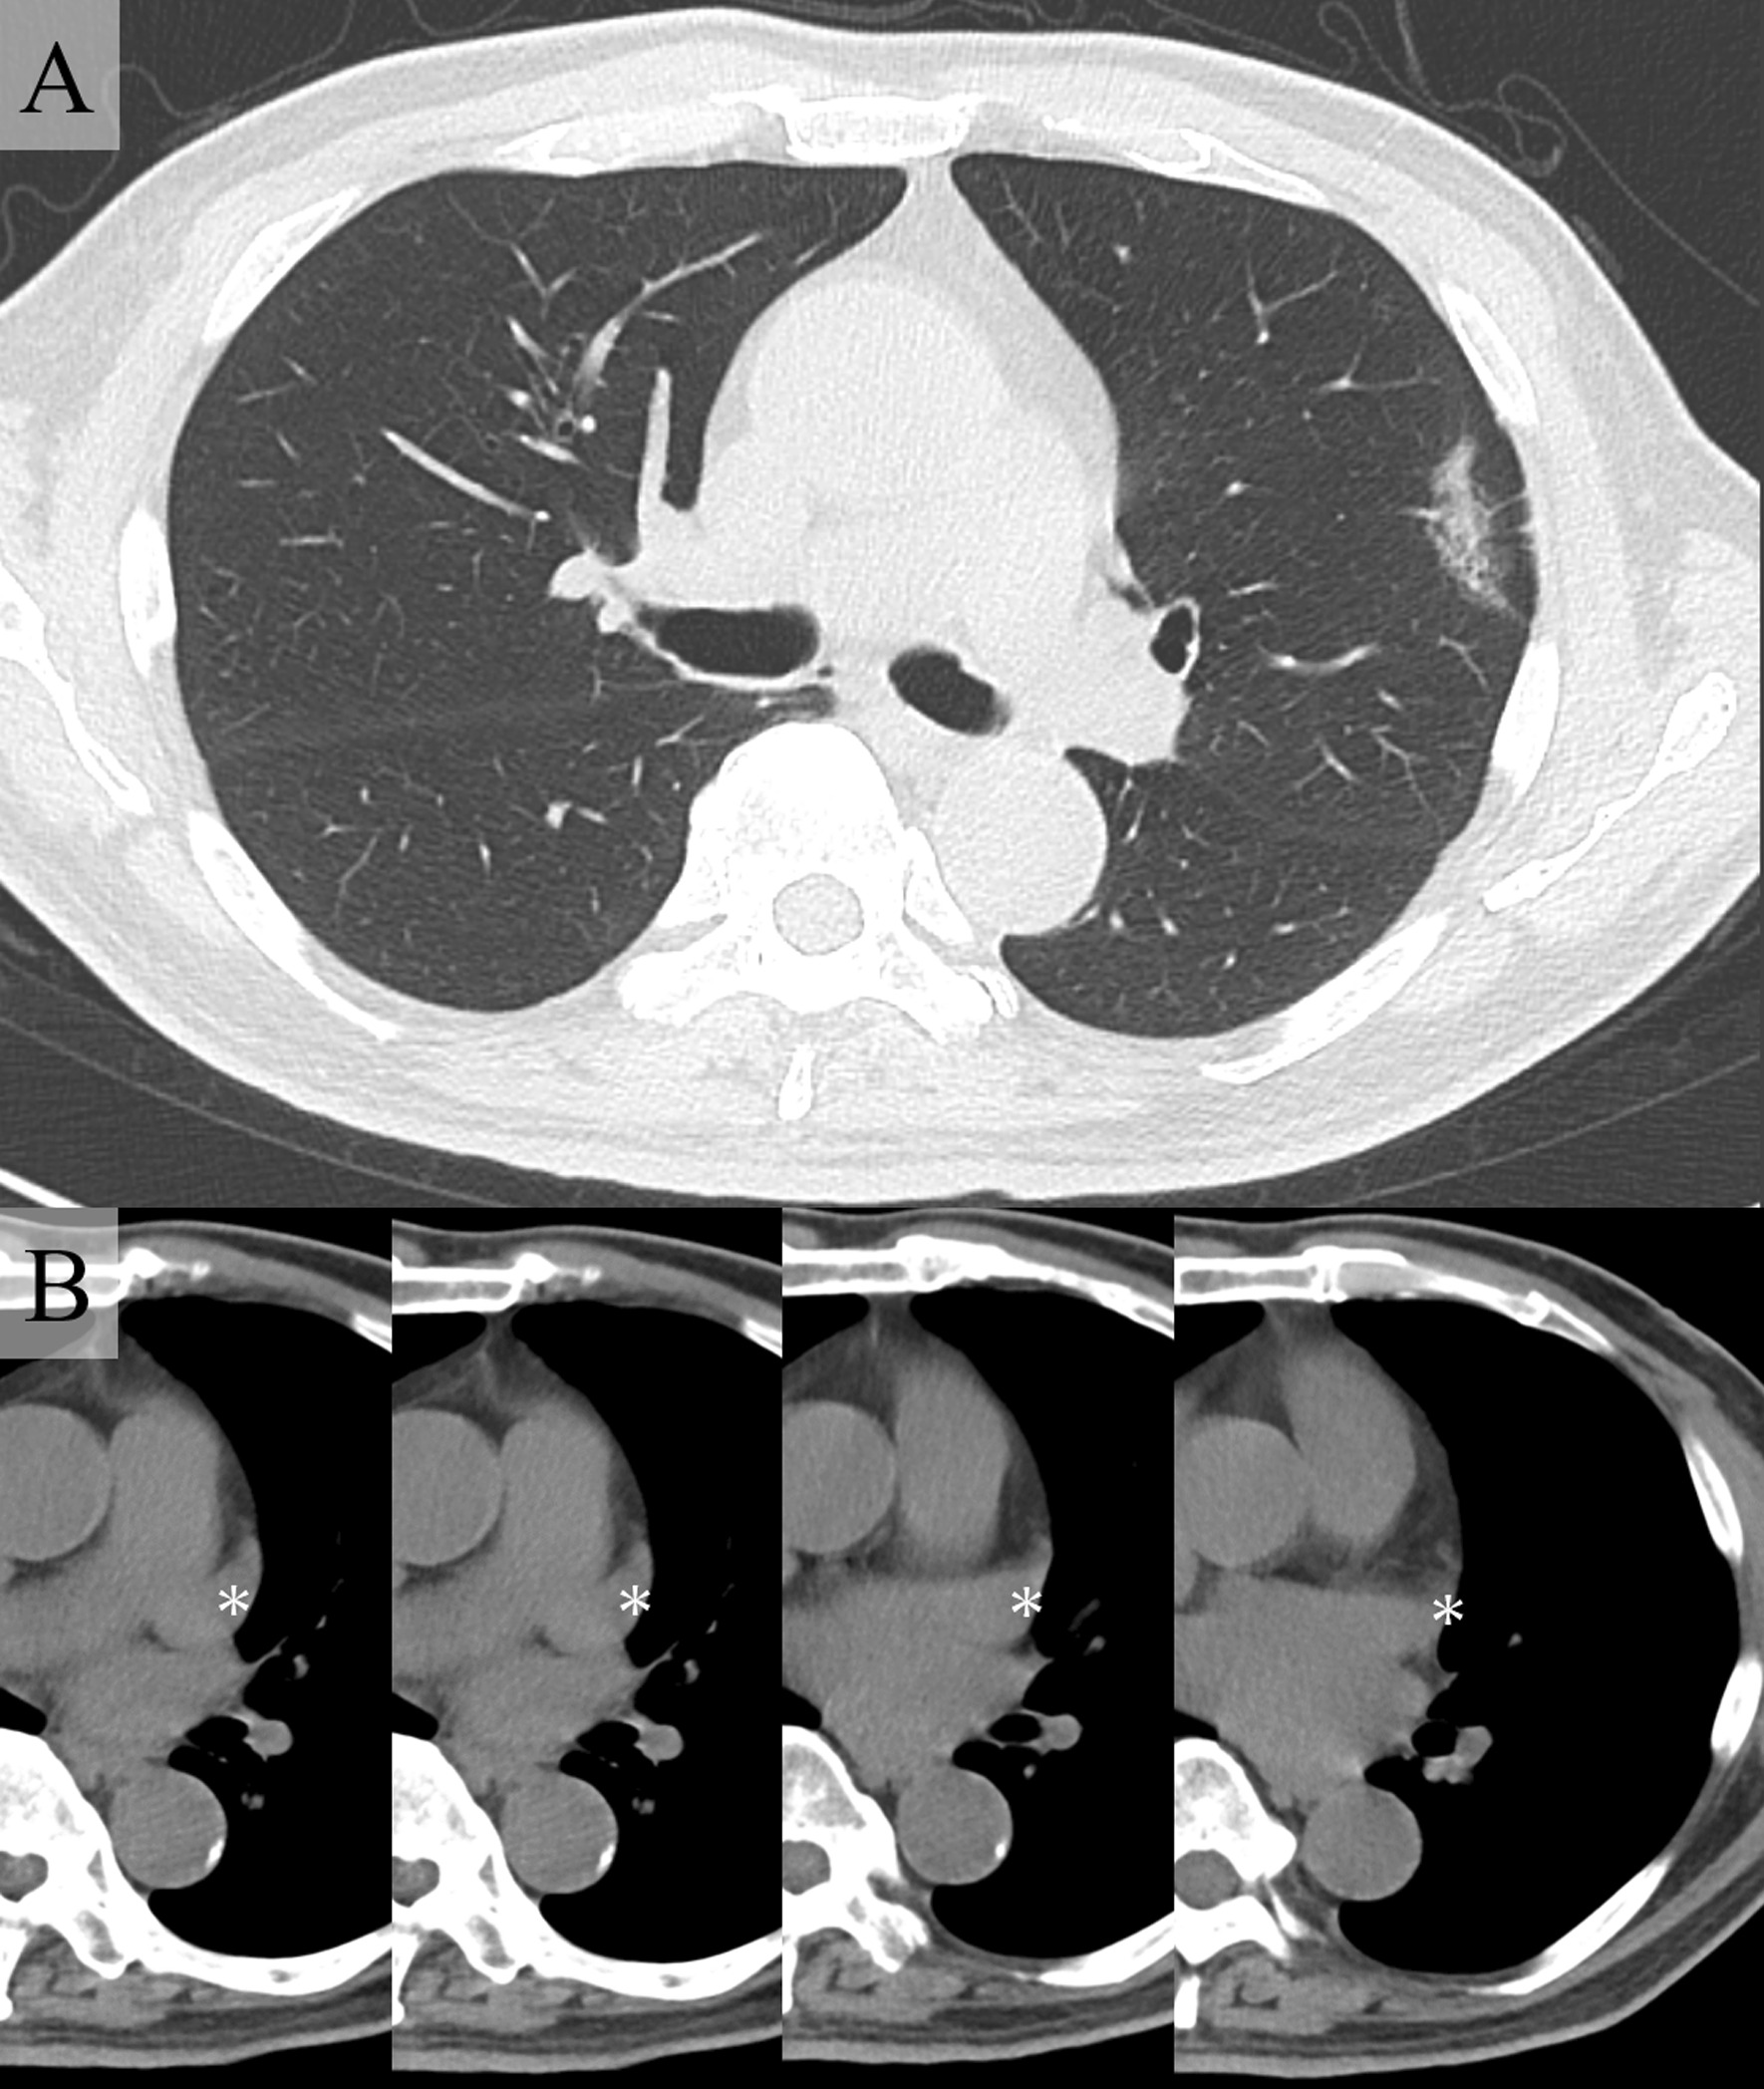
Computed tomography imaging. A A ground glass shadow with a maximum tumor diameter of 32 mm and a solid component diameter of 24 mm in the left lung S3. B No abnormal findings seen in the cardiac shadow (white asterisk indicates left atrial appendage)
